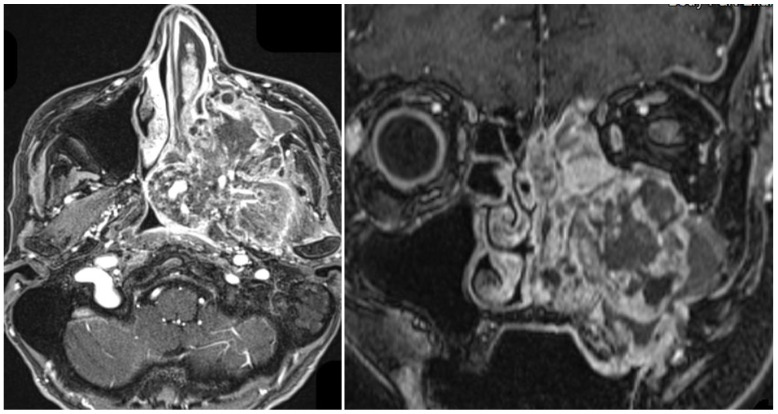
Contrast-enhanced MRI showing a large heterogeneous osteogenic osteosarcoma of the left nasal cavity and paranasal sinuses with extensive invasion of skull base and adjacent structures, arising 20 years after radiotherapy for nasopharyngeal carcinoma.
